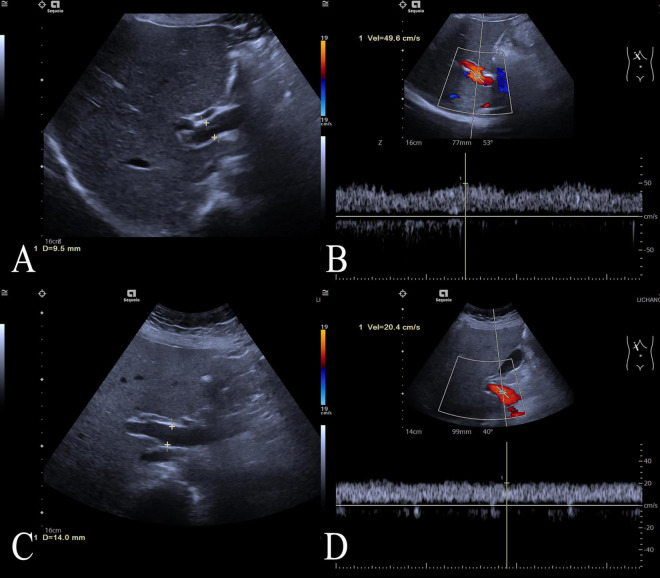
Two cases of portal vein measurement. Two cases of portal vein measurement using ultrasound imaging. (A) Ultrasound image of the control case showing a normal portal vein diameter measuring 9.5 mm, (B) and the case’s portal vein demonstrating a peak flow velocity of 49.6 cm/s; (C) Ultrasound image of an ALFP patient showing a wider portal vein diameter of 14.0 mm, (D) and the patient’s portal vein demonstrating a lower peak flow velocity of 20.4 cm/s.
